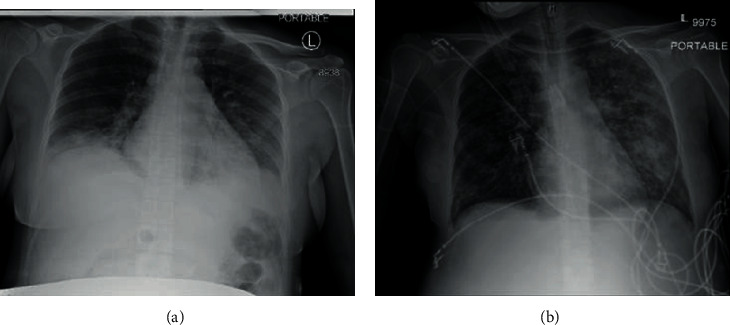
(a) Chest X-ray on admission, showing multifocal consolidative airspace opacities in lung bases bilaterally concerning multifocal pneumonia, possibly secondary to a viral pathogen. (b) Chest X-ray on HD 16 demonstrating generalized patchy bilateral lung airspace disease, with dense opacification and prominent interstitial lung markings representing the sequelae of ARDS.
